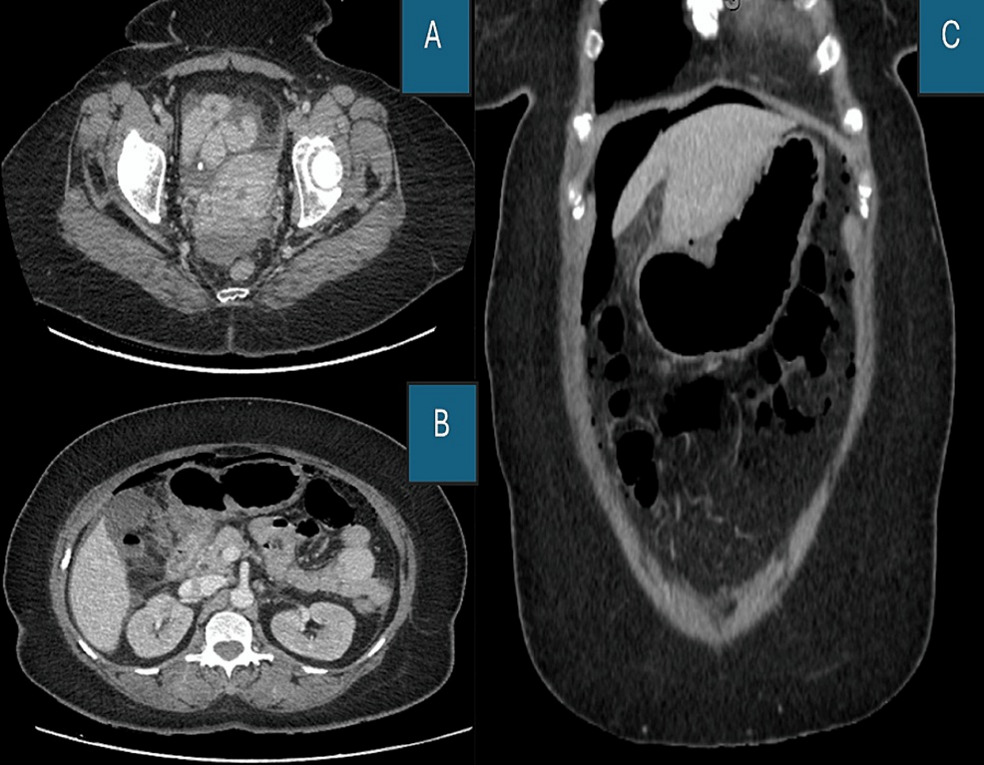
Abdominal CT with oral gastrograffin (A) CT axial cut showing mild pelvic fluids, (B) CECT axial cut of upper abdomen showing periduodenal mild fluids and oedema without contrast leak, (C) Abdominal CT coronal cut showing subdiaphragmatic perihepatic free air
